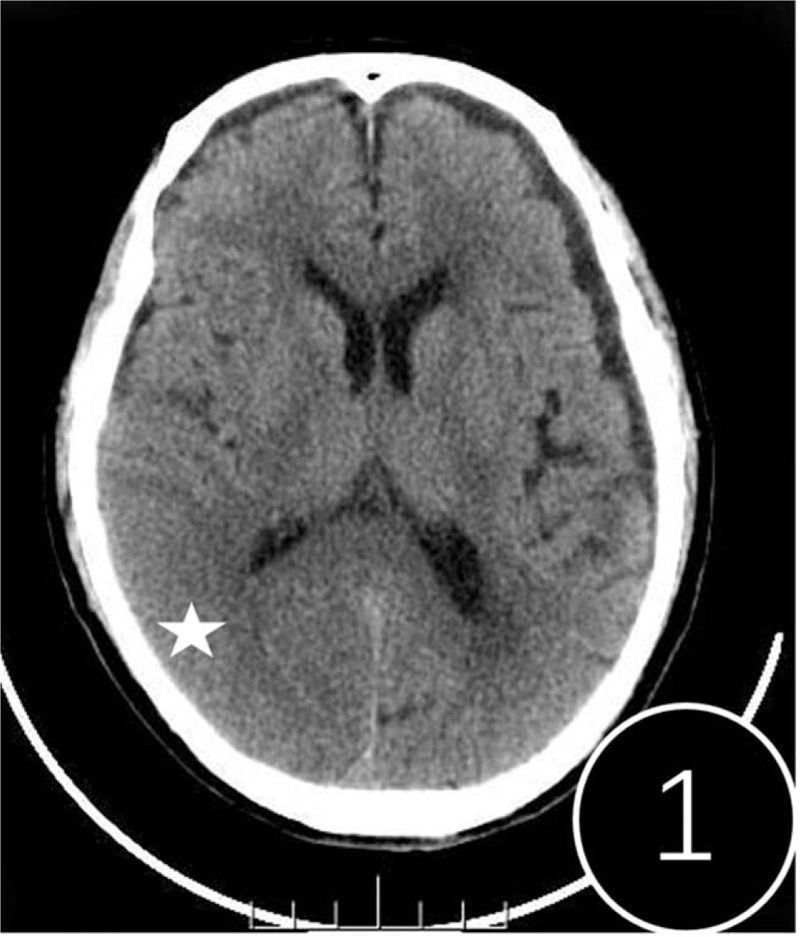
Brain computed tomography showed that the volume of the right temporal occipital lobe (pentacle) increased and the sulcus in the corresponding area became shallow and disappeared.
